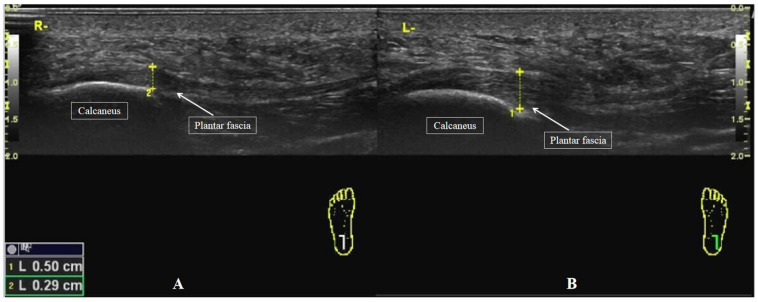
Individual example of a longitudinal sonogram of the PFT. The PFT was assessed at the anterior margin of the calcaneus. (A) The PFT of a healthy participant. (B) The PFT of a patient with PF.
